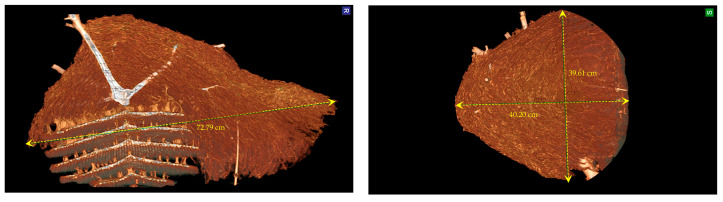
Asian hornet nest in different aspects and dimensions using CT scan, the patient orientation in space: (S)—superior and (R)—right.
